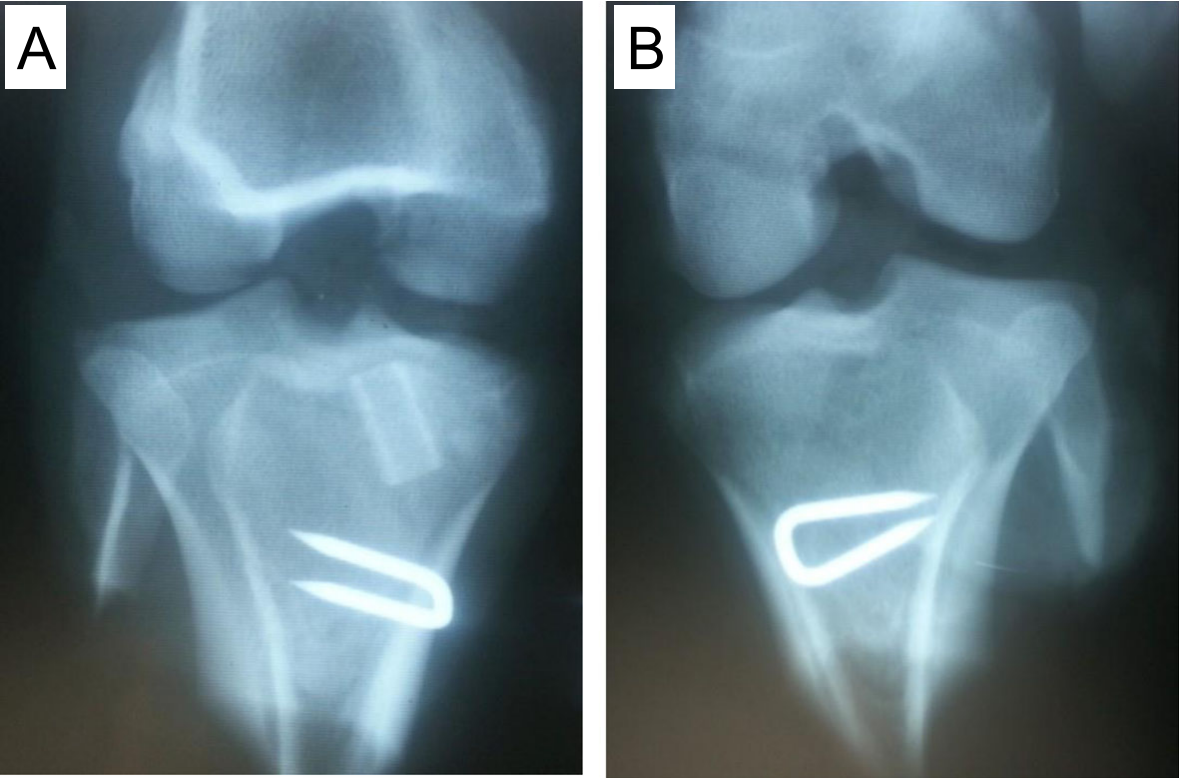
Bilateral anteroposterior radiographs of the knee after surgery. a TW (Titanium-web) limb. b Control limb
